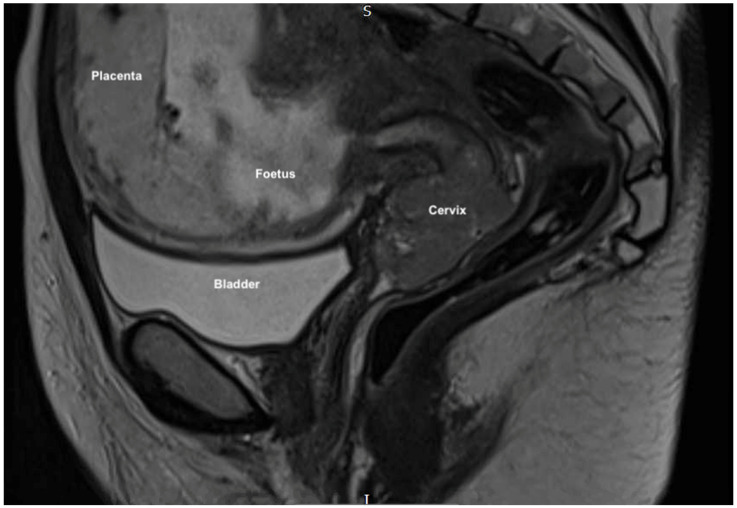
MRI sagittal scan showing the gravid uterus, anteverted and anteflexed, with a single foetus in cephalic presentation with cervical mass seen distally.
